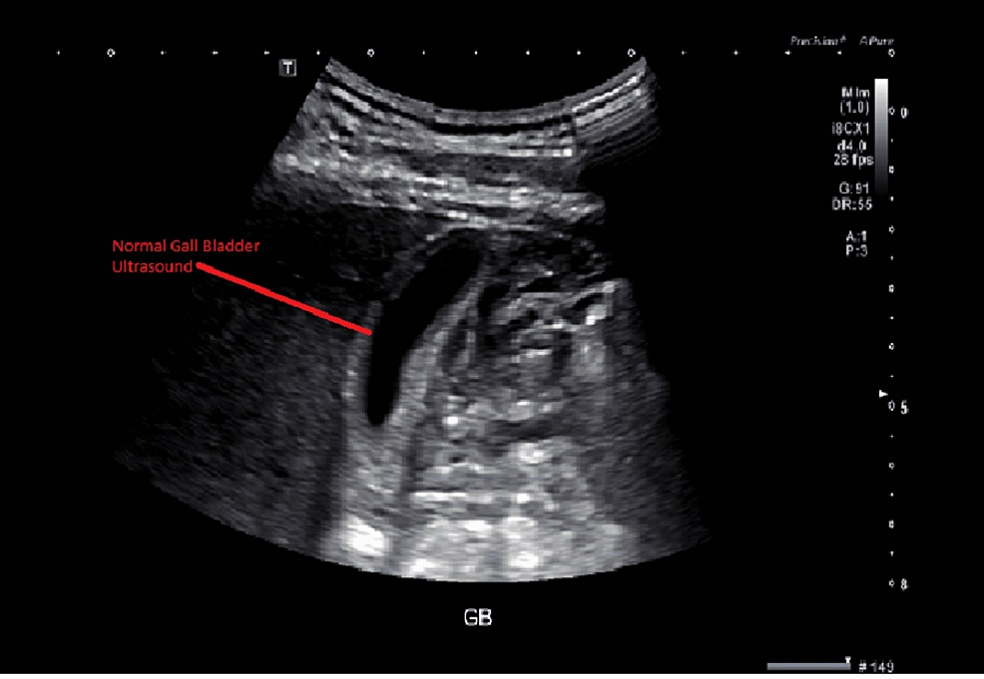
Gallbladder ultrasound: normal
